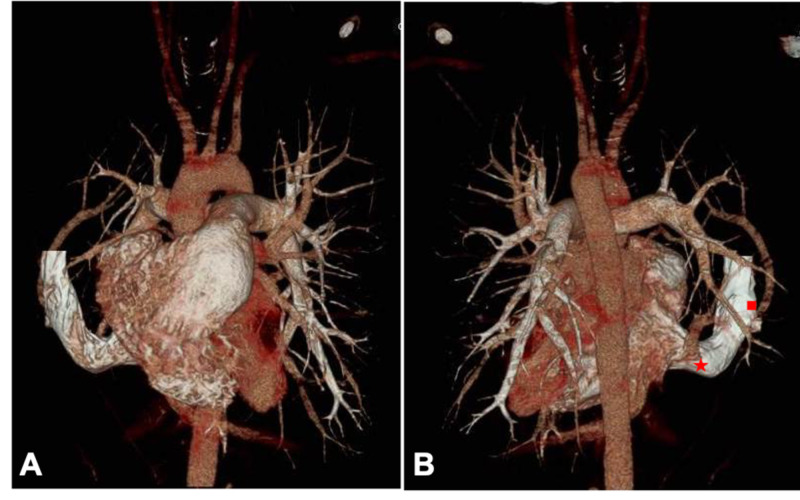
A three-dimensional reconstructed CT scan image of the heart. (A) The anterior view of the anomalous superior vena cava inserting into the right atrium with the inferior vena cava. (B) The posterior view of the anomalous superior vena cava. The large right lower lobe anomalous pulmonary vein (star) and right upper lobe pulmonary vein (square) can be seen inserting into the anomalous superior vena cava.
